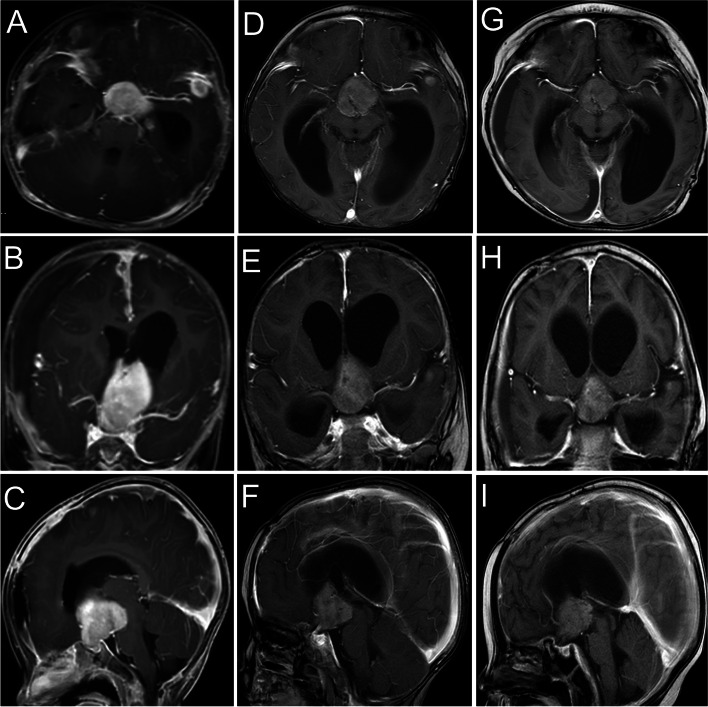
The MRI of the head during chemotherapy with the measurement of RECIST (The Response Evaluation Criteria In Solid Tumors). Compared with the lesions (A-C) after resection (2.4 × 3.1x2.6 cm), the lesion on MRI was stable two months after chemotherapy management with crizotinib and vincristine (D-F) (2.6 × 3.3x2.8 cm). After two months of treatment with cytarabine, crizotinib, and vincristine, tthe effect of the treatment was partial response (G-I) (2.4 × 2.3x2.1 cm). The measured resolution for images was 360 ppi
